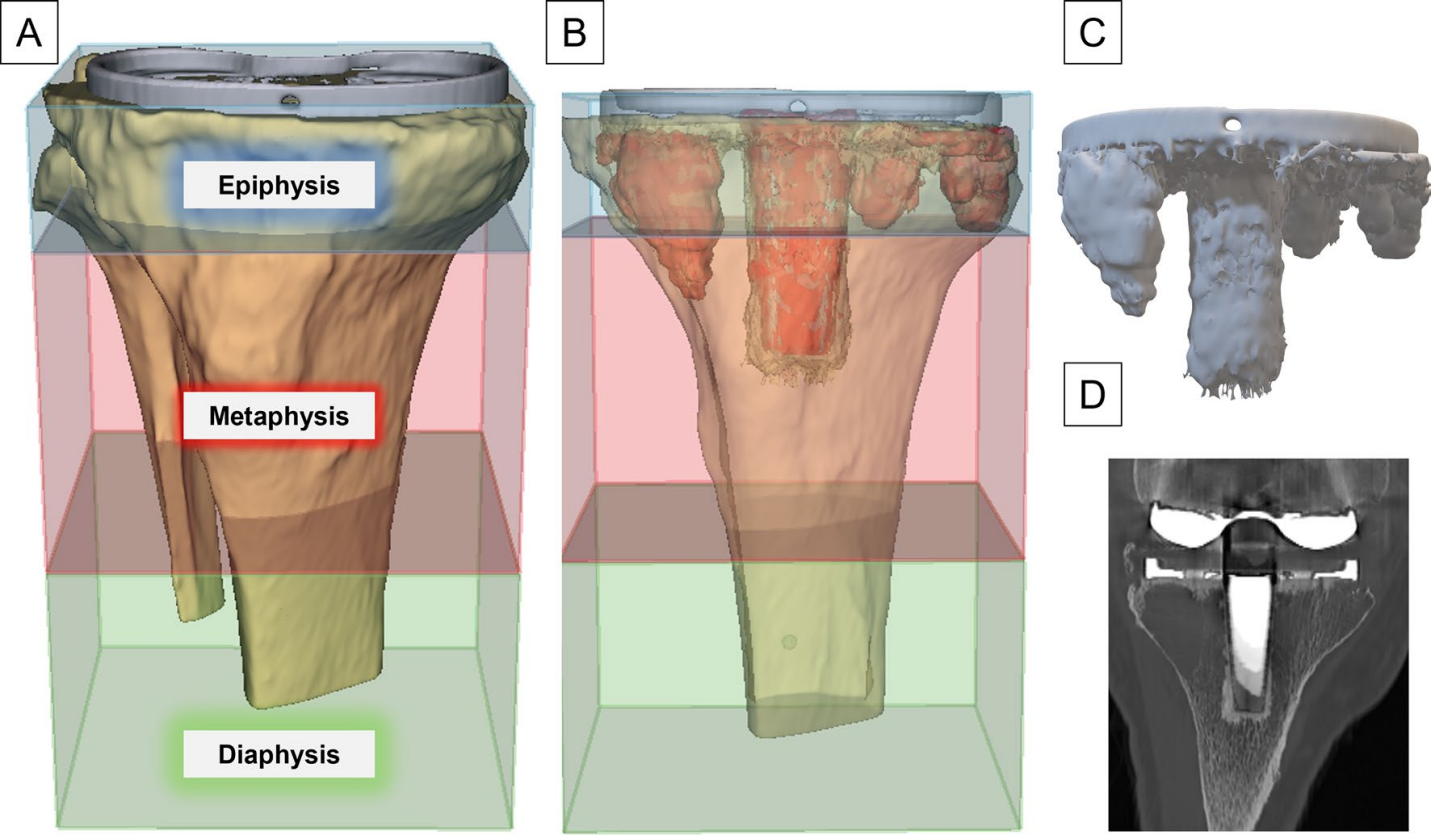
Three-dimensional segmentation of tibial bone defects. A Tibial epiphysis (blue) and metaphysis (red) are defined by a square with a side-length that is the same as the widest part of the tibial epiphysis. Bone outside of this square is considered to be diaphysis (green). B To determine the volume of the remaining tibial bone after removal of the prosthesis, the defect was subtracted from the tibial bone. C Segmented 3D model of the defect. D Coronal plane of the respective CT
